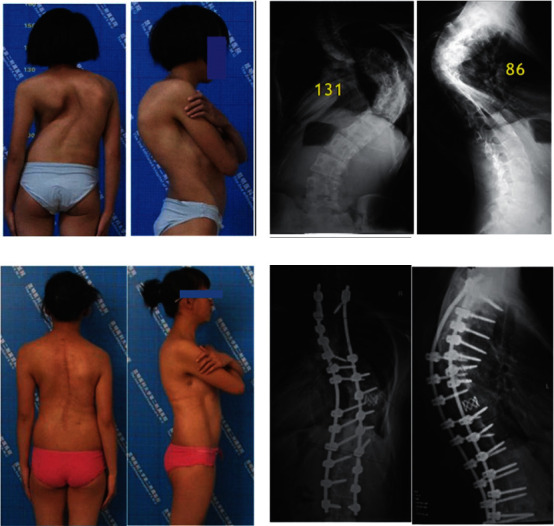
A 17-year-old girl with thoracic kyphoscoliosis. (a) Clinical feature preoperative. (b) X-ray of anterior-posterior and lateral preoperative. (c) Clinical feature at 2 years follow-up after PVCR. (d) X-ray at 2 years follow-up.
